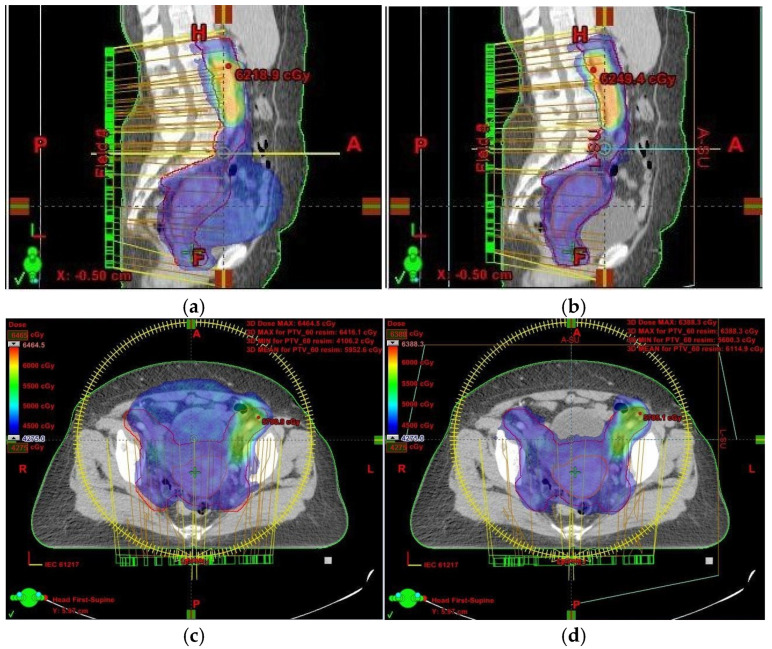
(a–d) CT scan treatment planning for the initial and rescan plan—axial and sagittal views: comparison of the 95% isodose curve from the PTV (blue)—left (a,c): initial plan; right (b,d): rescan plan. PTV margin is indicated in red. On the left, the original irradiated volume (blue) significantly exceeds the updated PTV post-fluid removal, leading to unnecessary exposure of healthy tissue.
